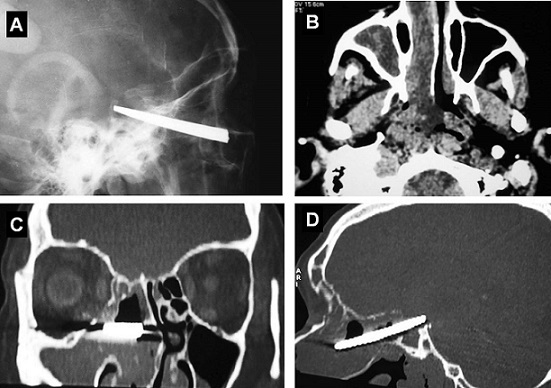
Metallic foreign body from the nasal cavity to the sella ((A), X-ray profile of the skull). Scano-graphic aspects: Filling reaction speed of the maxillary sinus and the ipsilateral nasal cavity with an inflammatory granuloma choanal area and retro-choanal right in axial section ((B) parenchymal window), coronal reconstructions (C) and sagittal (D) (bone windows)
